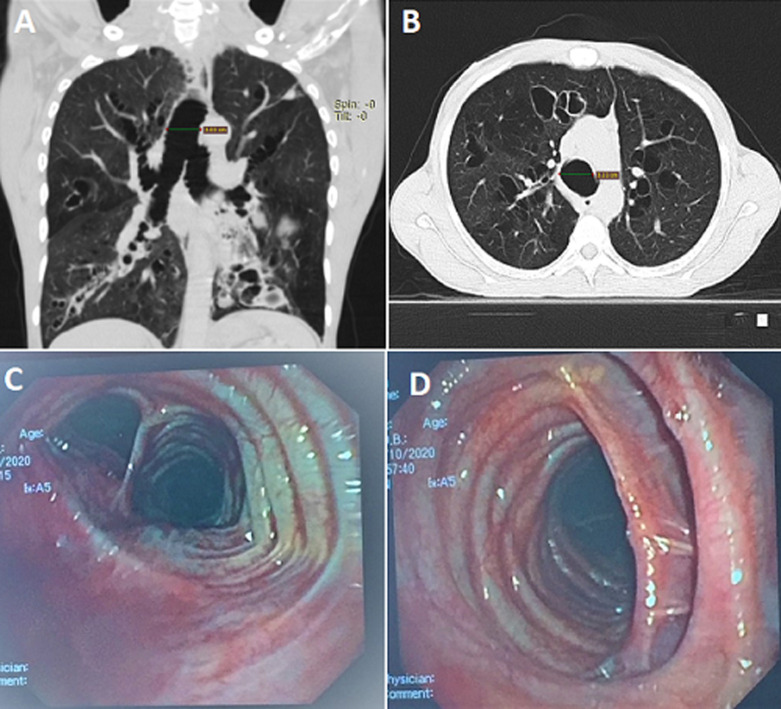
(A,B) chest CT showing a dilated trachea (>3cm), dilated main bronchi, and cystic bronchiectasis, mainly in the lower lobes; C) bronchoscopy image showing a significantly dilated trachea and bronchi; D) tracheal diverticula
